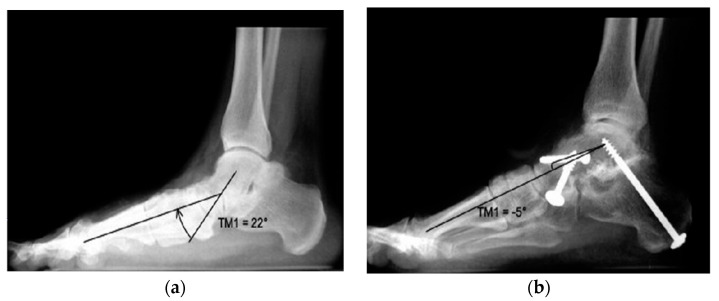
Lateral weight-bearing radiographs demonstrating the talo–first metatarsal (Talo-M1) angle. Preoperative view (a) and postoperative view at final follow-up (b).
